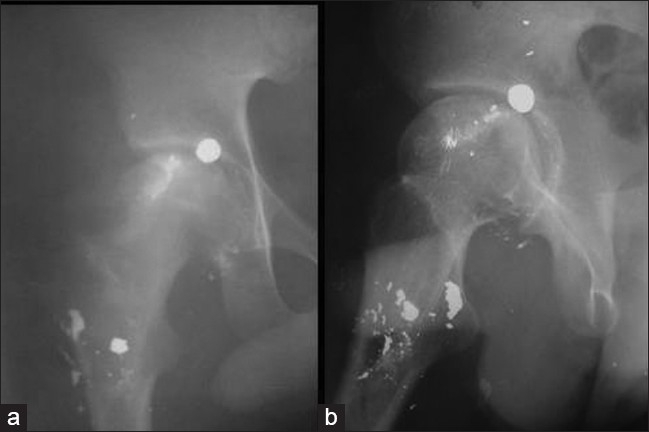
X-ray (anteroposterior and lateral views) of hip joint showing bullet in the acetabulum
